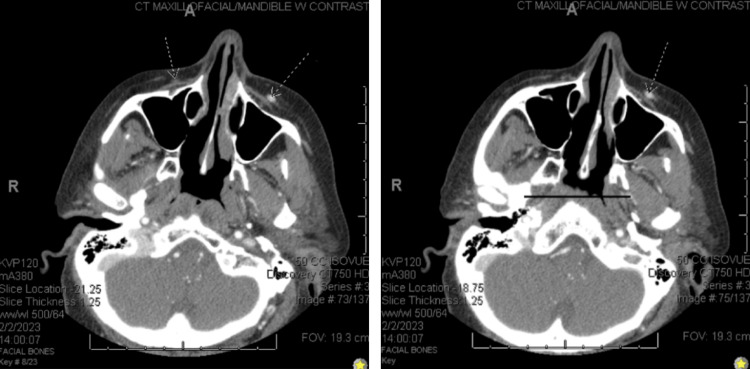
Two slides from the patient's CT with contrast of the maxillofacial/mandibles demonstrate a diffuse asymmetric prominence of the left facial vein when compared to the right facial vein. This indicates the presence of a varix on the left.
